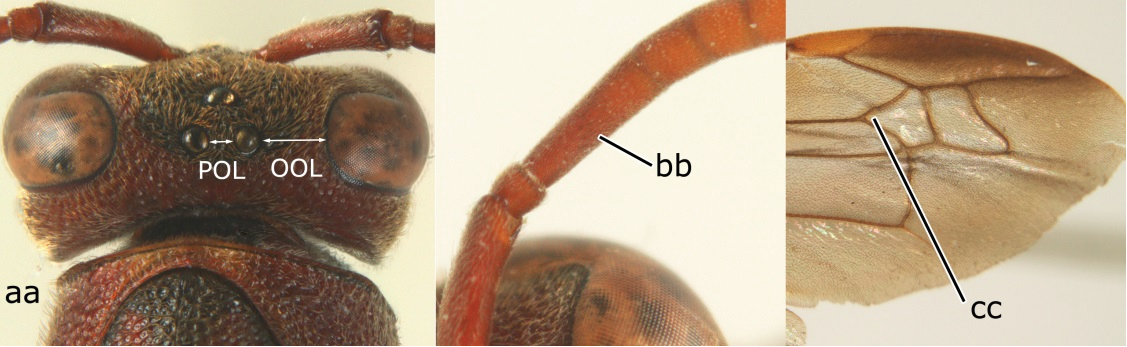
5	Pronotal carina nearly straight (a); propodeum distinctly obliquely striate (b); propodeal valvula large, nearly circular (c); first metasomal tergite comparatively long, with petiolus parallel-sided, widened part swollen submedially and narrowed near apical margin (d); second metasomal segment oblique apically, with tergite longer than sternite (e); male antenna with distinct tyloids, apical two thirds of apical flagellomere excavated and curved (f)	*Ropalidia fasciata* (Fabricius, 1804)
